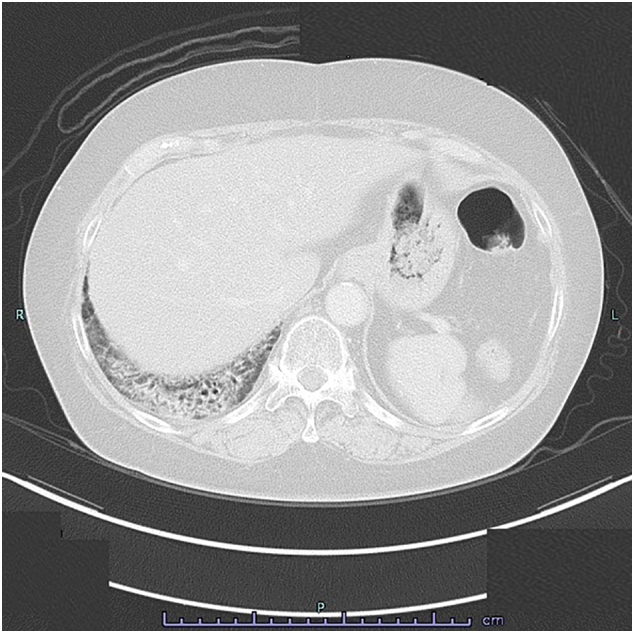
Contrast-enhanced CT examination revealed interstitial pneumonia developed after nine courses of nivolumab.
